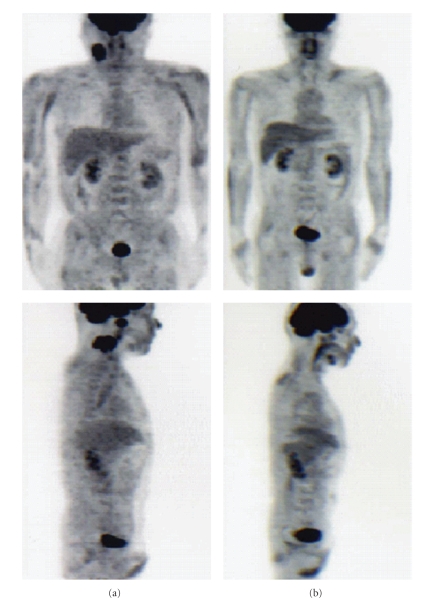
PET-CT. (a) Pre-treatment. Intense FDG uptake is detected in the left cervical region and the nasopharynx. Mild FDG uptake is detected in the muscles of the entire body, especially the upper extremities. (b) Post-treatment. FDG uptake with residual tumor is not detected. FDG uptake in the muscles is improved compared to pre-treatment.
